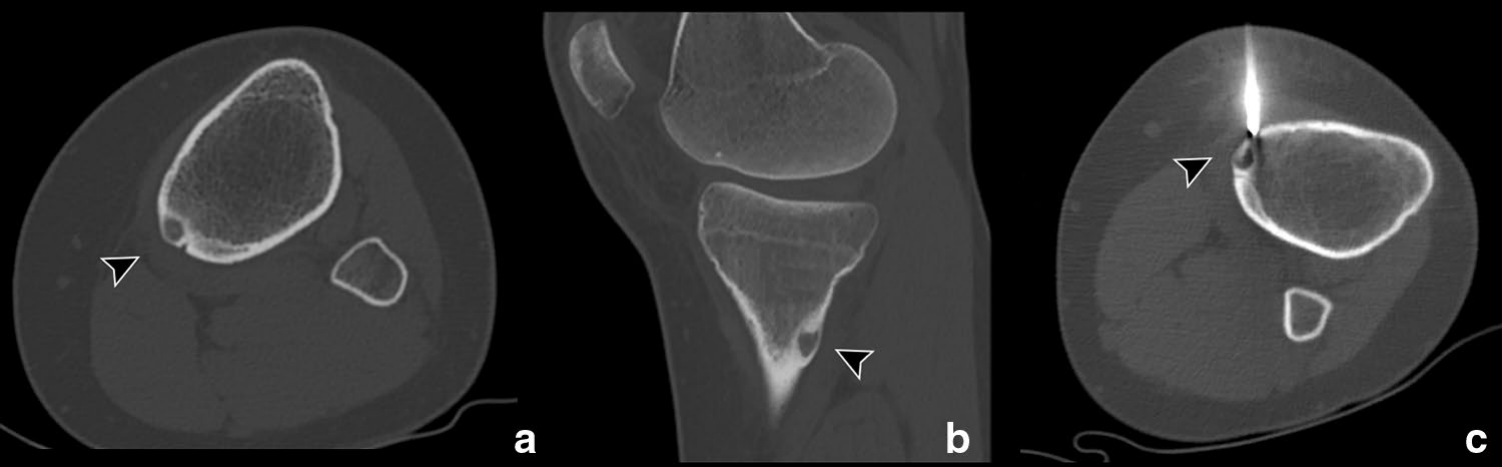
Osteoid osteoma of the left tibial diaphysis. Axial (a) and sagittal (b) CT scans show the intracortical radiolucent nidus with a central region of mineralization (arrowhead). c Axial CT scan performed during RFA procedure shows bone drill/biopsy kit over the cortical surface approaching the nidus
